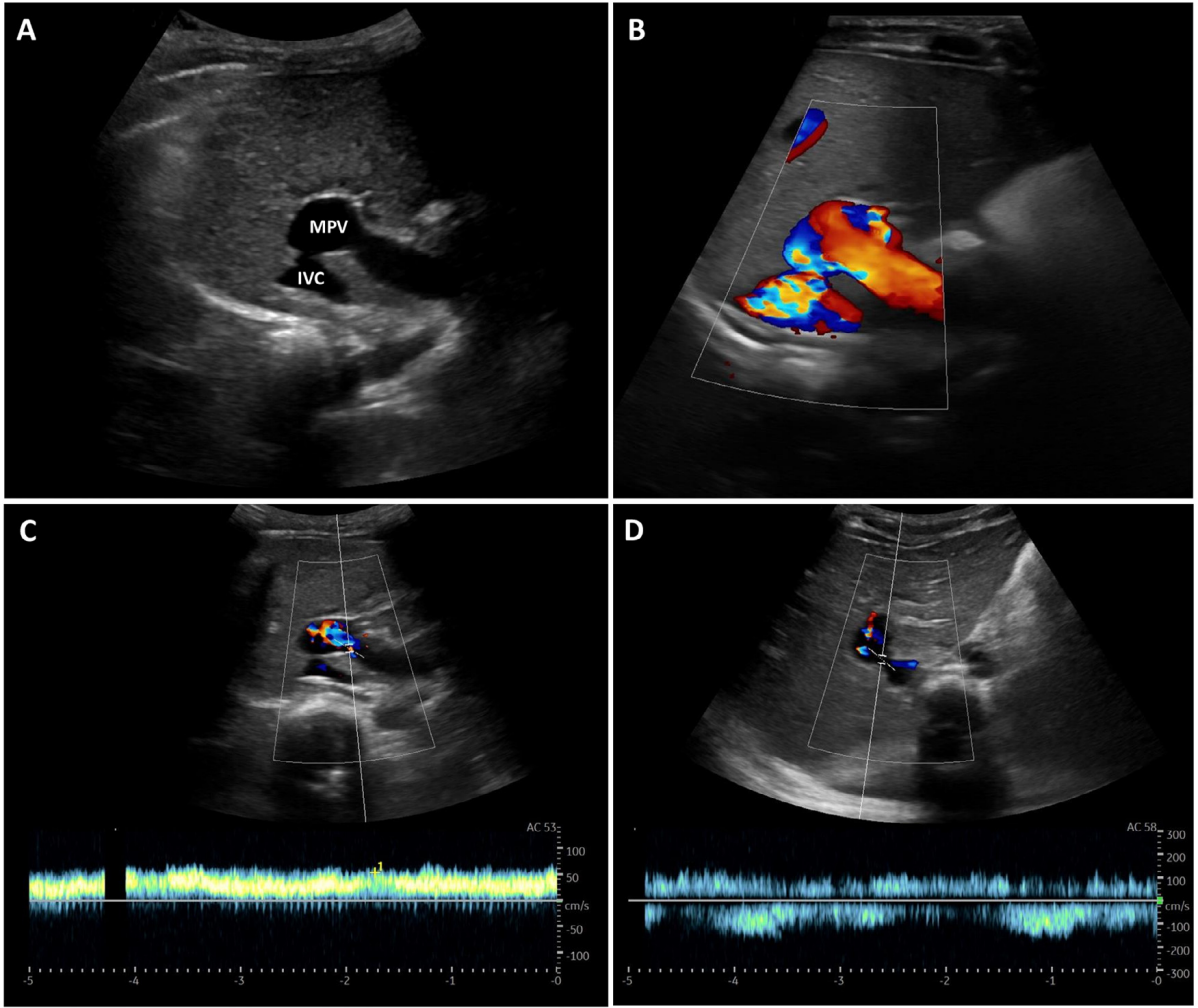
Ultrasonography with color and spectral Doppler. (A and B) Ultrasonography and color Doppler show an abnormal communication with flow between the MPV and the IVC. The MPV is enlarged, with a maximum diameter of 18 mm. (C) On spectral tracing the MPV has a normal monophasic and hepatopetal flow, but with high velocity (medium velocity of 54.9 cm/s). (D) Spectral analysis of the shunt depicts alternating hepatopetal and hepatofugal flow. IVC, inferior vena cava; MPV, main portal vein.
